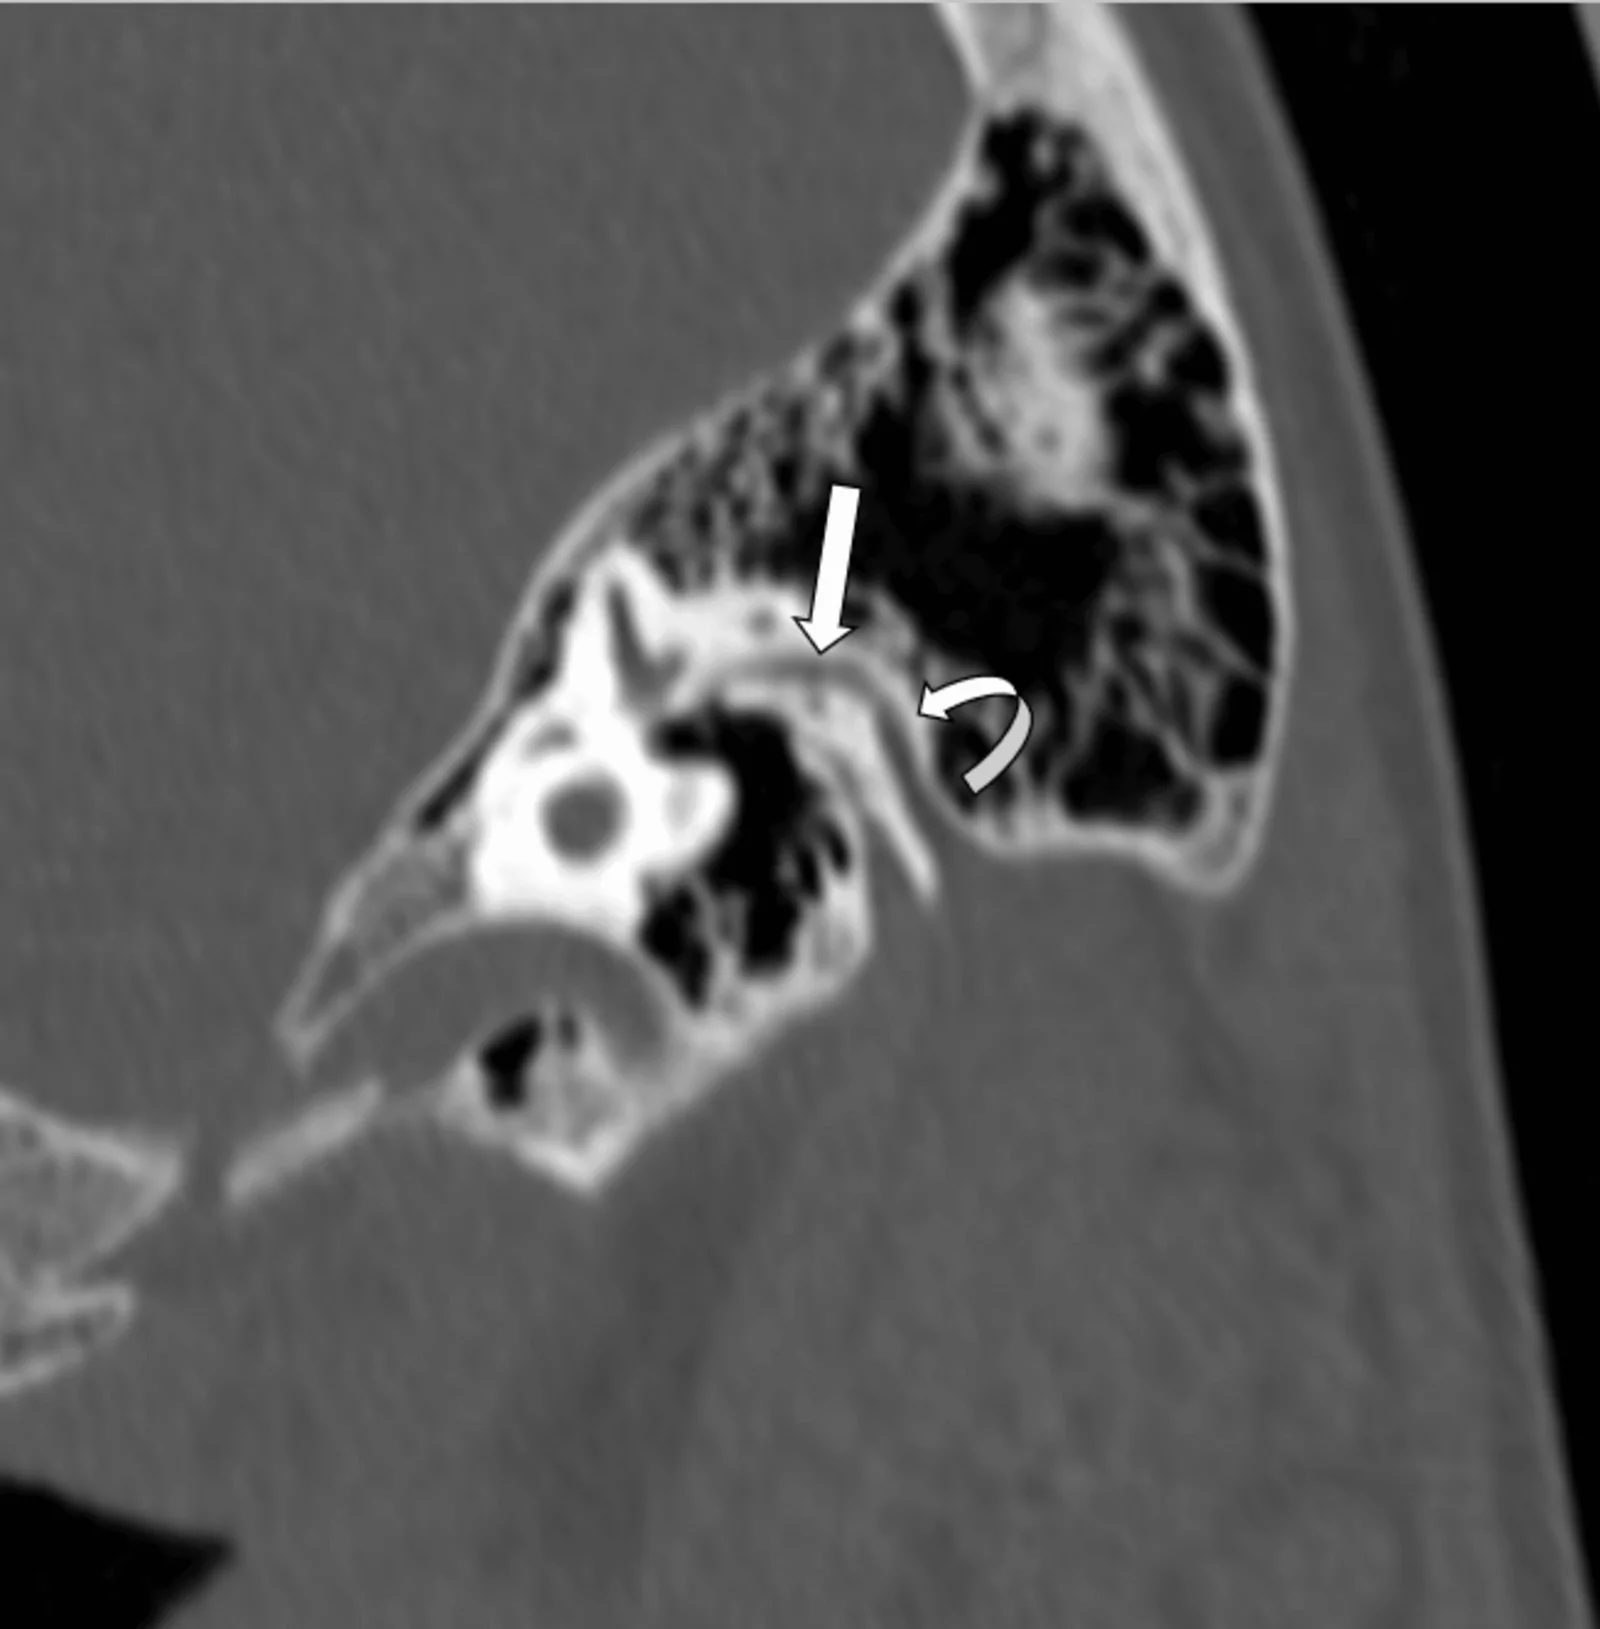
High-resolution computed tomography (HRCT) of the left temporal bone - oblique sagittal section The images demonstrate a normal, well-defined bony facial canal for comparison. Straight arrow: tympanic part of the facial nerve canal; curved arrow: mastoid part of facial nerve canal
